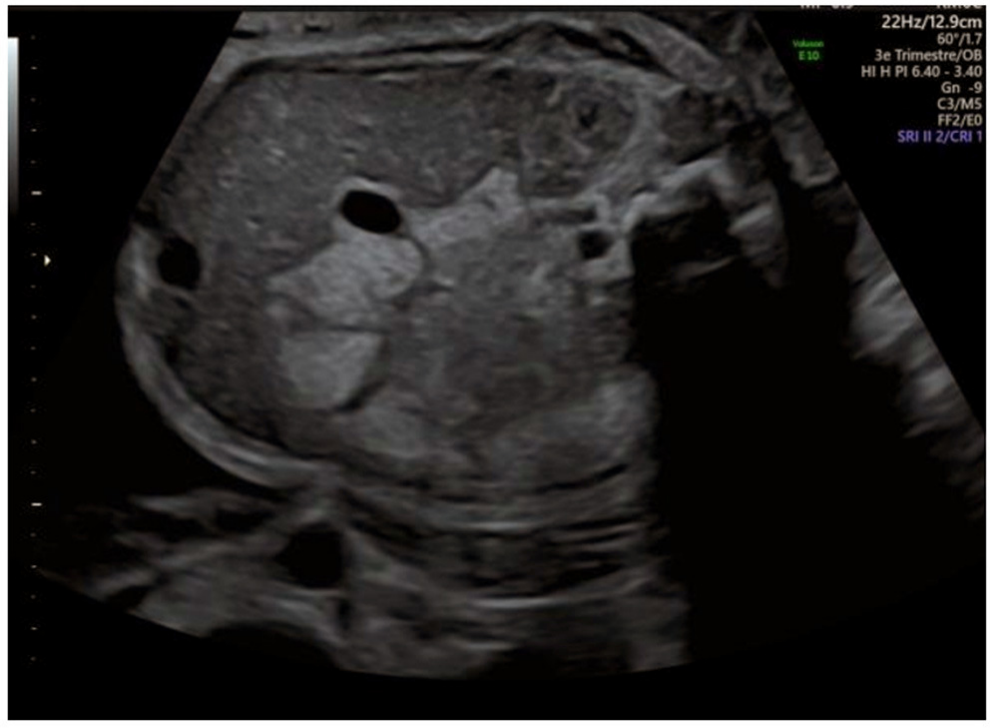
Antenatal ultrasound at 30 weeks of gestation.
